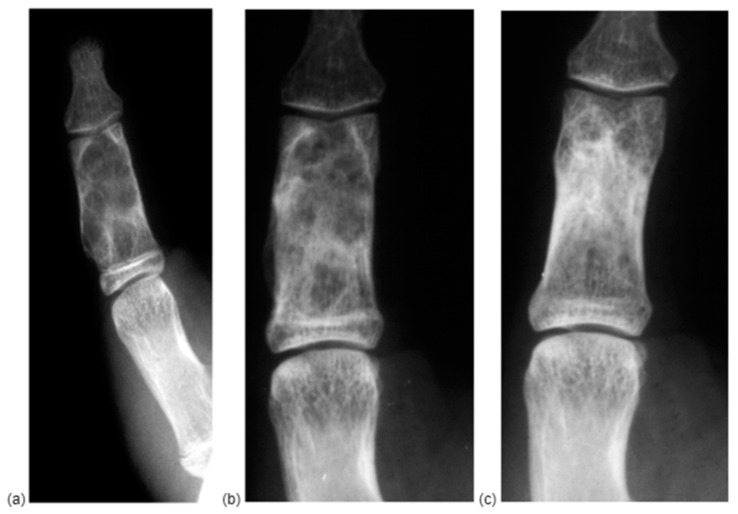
X-RAY of a P2D2 enchondroma at first diagnosis (a), at 2 months (b), showing a fast reconstruction of the cancellous bone with a slower remodeling of the normal morphology of the bone, which was obtained at 12 months (c).
